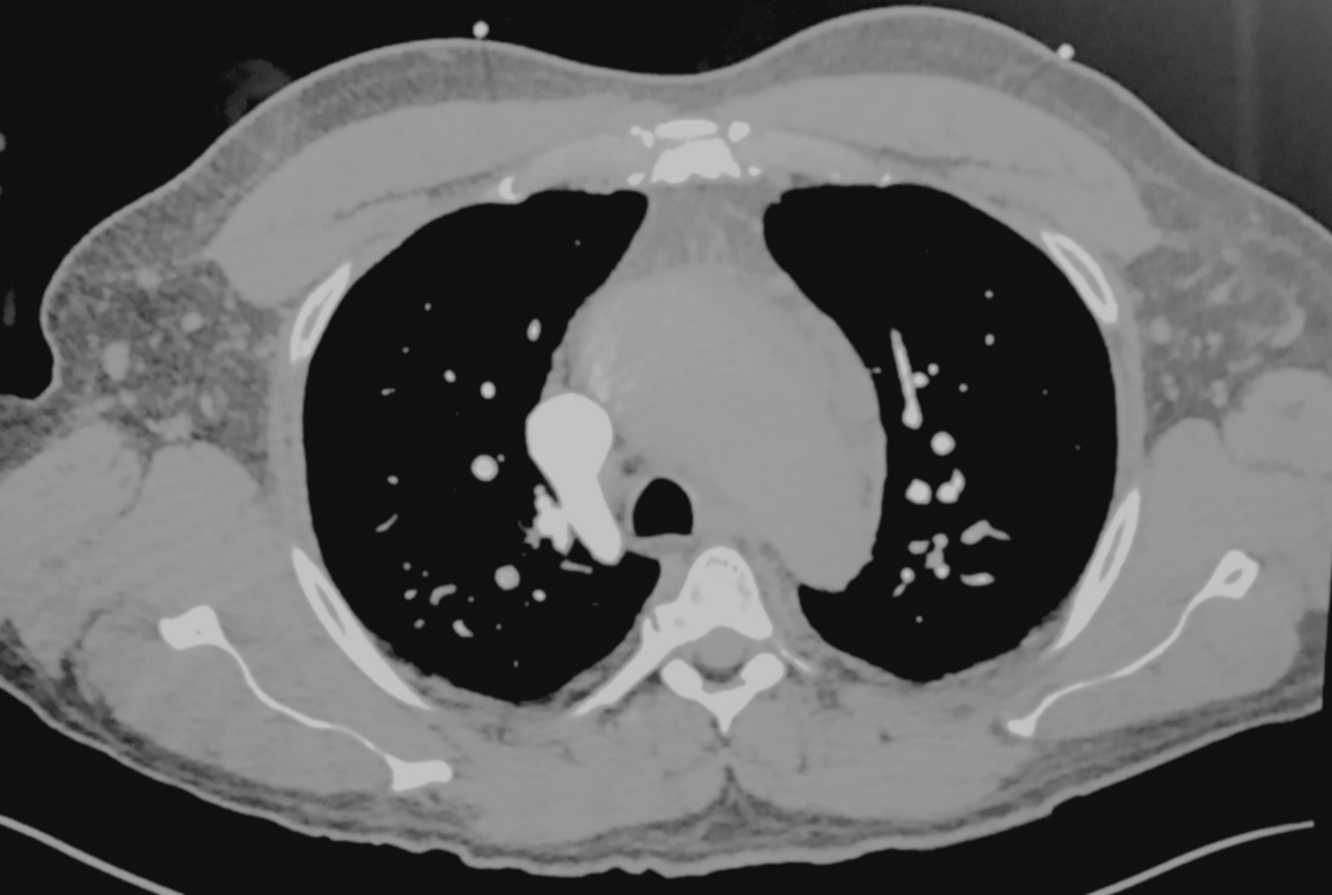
Initial transverse computed tomography (CT) lacking signs suggestive of acute aortic dissection
